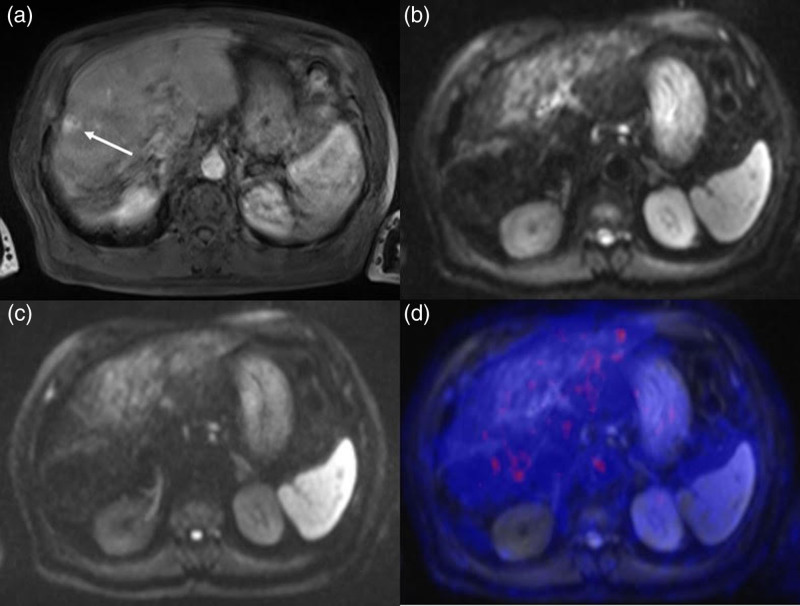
Contrast-enhanced MRI showing a hypervascular hepatic lesion in arterial phase (arrow), suspect for hepatocellular carcinoma (HCC) recurrence (Fig. 4a), but the lesion showed no diffusion restriction nor pathological hypermetabolism on fused PET/MRI images (b–d). Follow-up imaging confirmed that the lesion, which was classified as an arteriovenous fistula, was stable in size.
